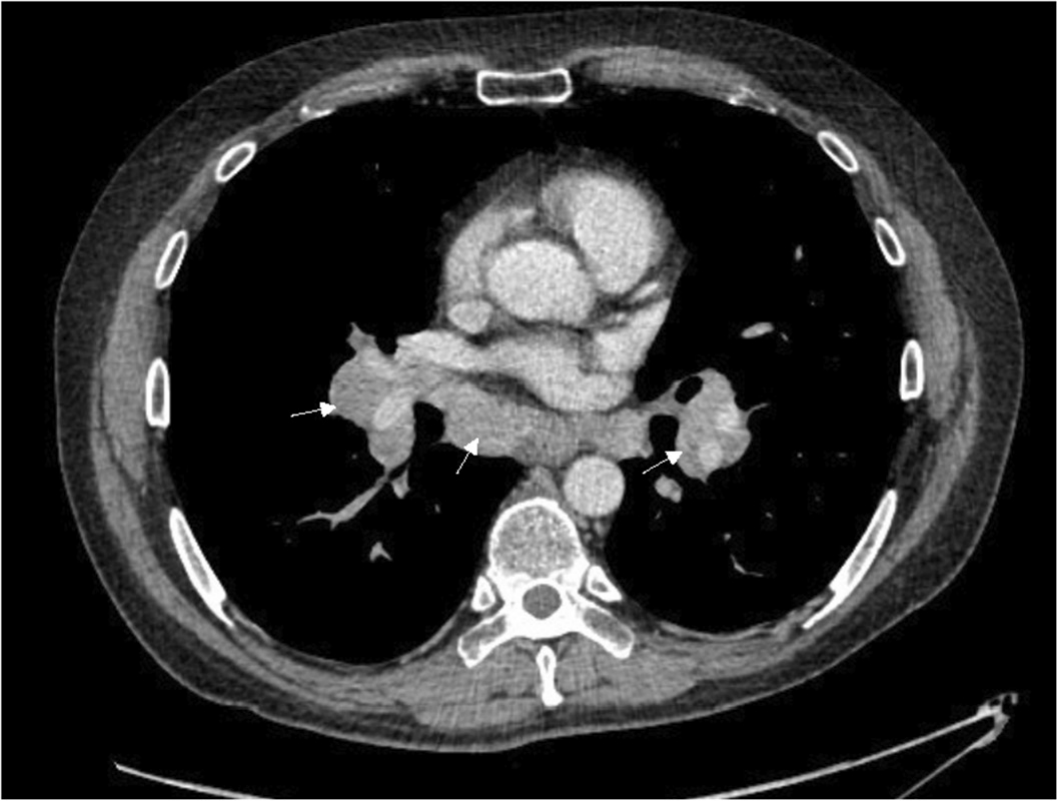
Enhanced CT scan of the chest demonstrating bilateral hilar and mediastinal lymphadenopathy (indicated by arrows).
